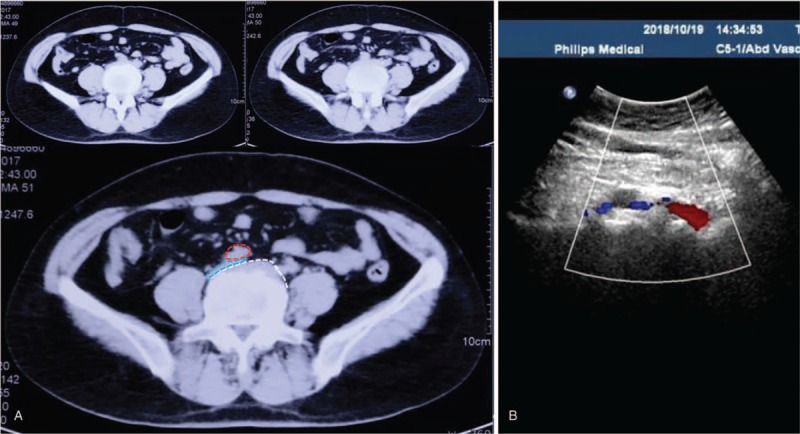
Preoperative CT shows the anterior LDH on the segment of L4-5, which causes the stenosis of inferior vena cava (A). The right iliac common artery is outlined by red, the LDH is outlined in white, and the compressed inferior vena cava is outlined in blue. Preoperative abdomen Doppler ultrasound shows the over 50% patency loss on the left inferior vena cava (B). CT = computed tomography, LDH = lumbar disc herniation.
